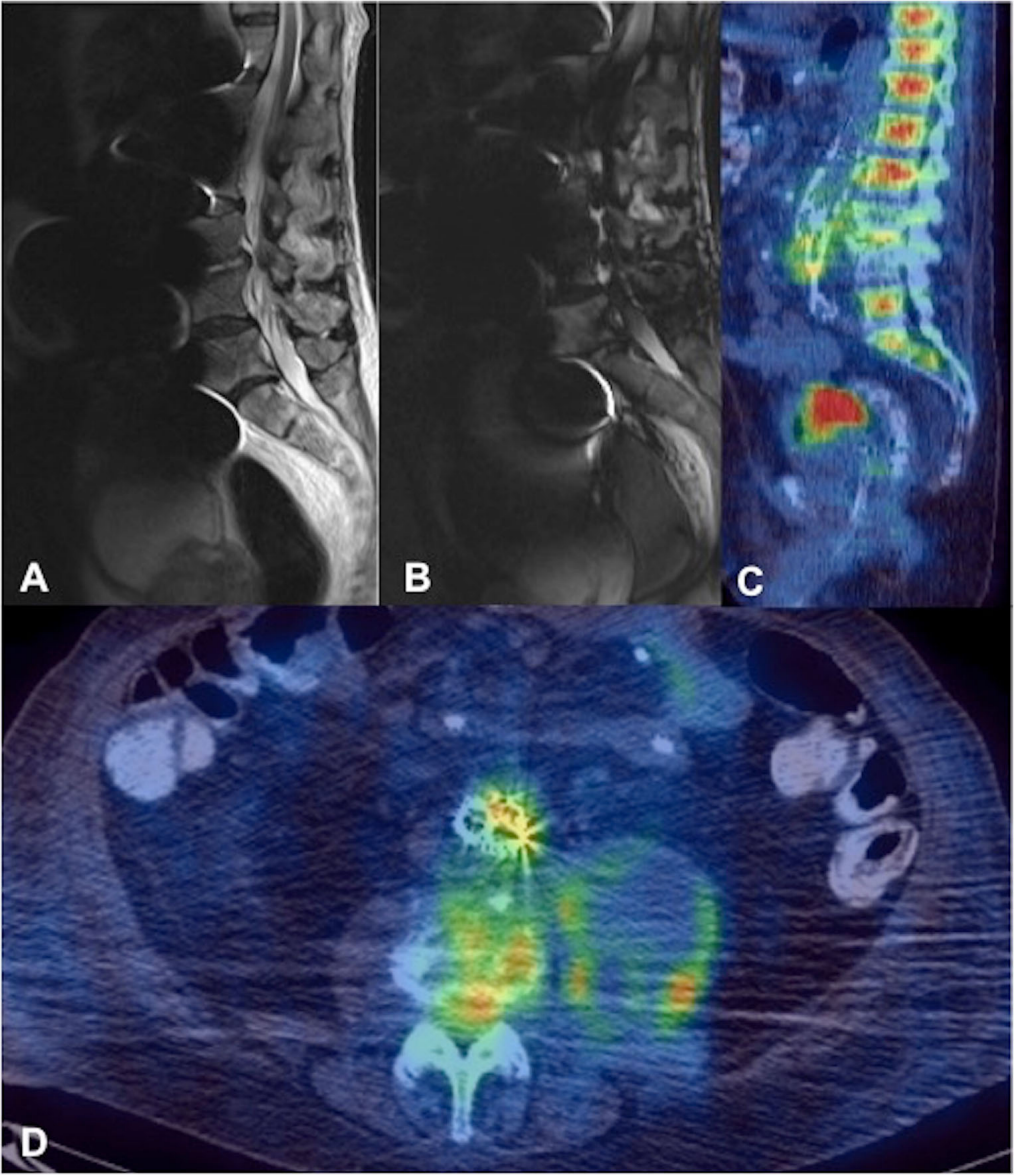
A 75-year-old man (patient 2). a Sagittal T2 and b STIR sequences demonstrating marked susceptibility artefact limiting image interpretation. Increased signal can be seen in the L3/4 disc in a, but no assessment of the paravertebral soft tissues can be made. c Sagittal and d axial PET/CT demonstrate abnormal increased uptake related to the EVAR stent graft with soft-tissue density and increased uptake extending into the L3/4 disc space, where there is endplate erosion and loss of disc height consistent with spondylodiscitis. Expansion of the left psoas with peripheral uptake suggests associated psoas abscess formation. Diffusely increased marrow uptake elsewhere in the spine was thought to be reactive
